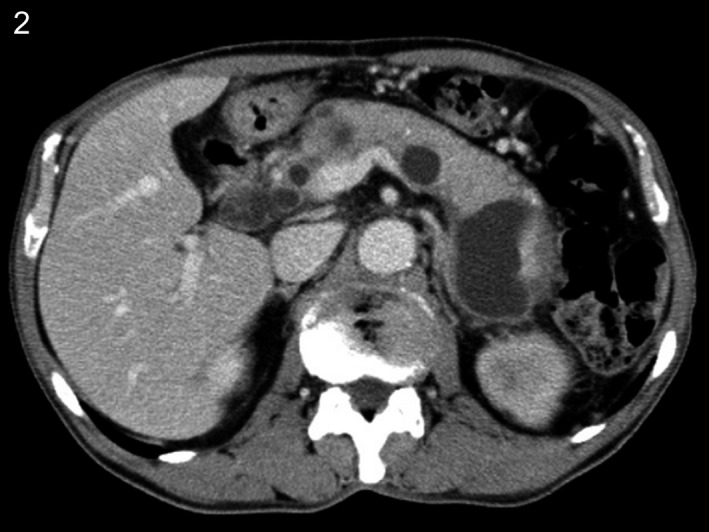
Computed tomography showed diffuse swelling of the pancreas, multiple cysts from the head to the tail, and calcification
